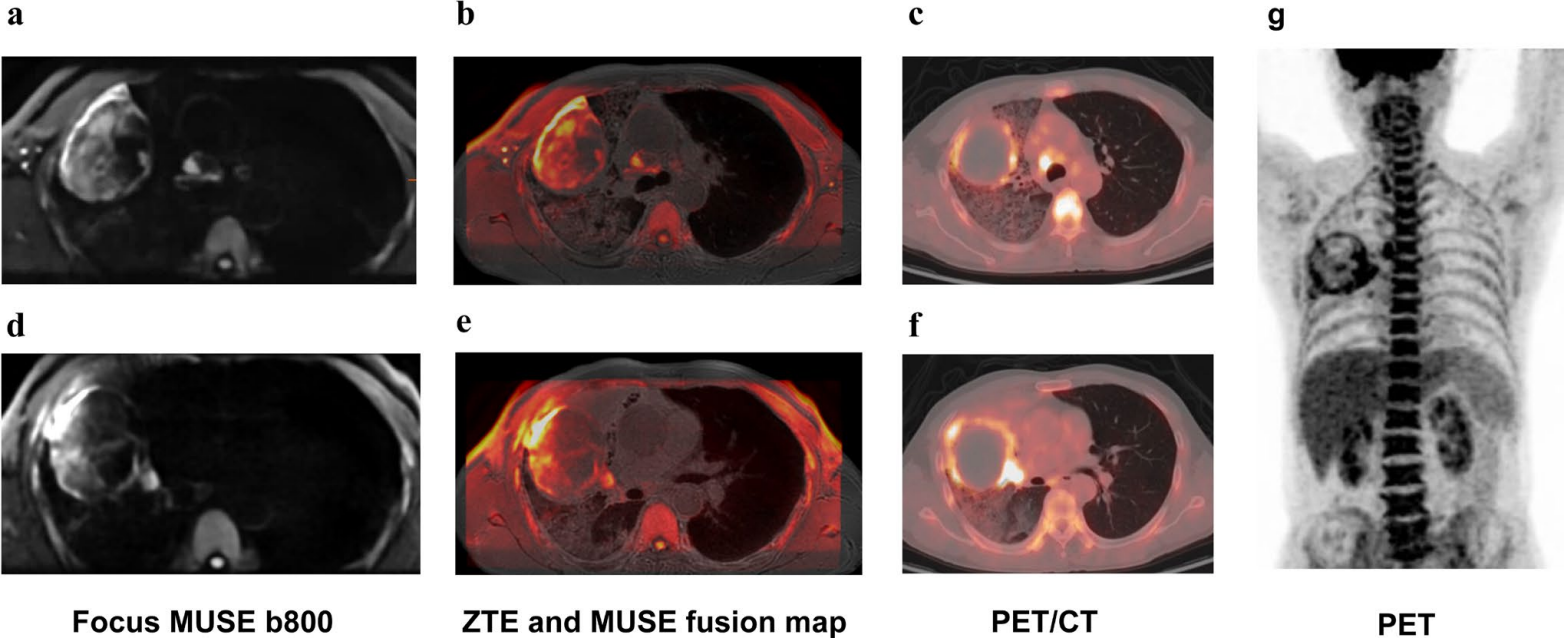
Male, 53 years old, lesion in the upper lobe of the right lung, compression atelectasis of the adjacent lung tissue, localized solid lesions, and enlarged lymph nodes visible in the mediastinum. (a, d) FOCUS MUSE DWI (*b* = 800); (b, e) ZTE and MUSE DWI fusion maps; (c, f, g ) 18F‐FDG PET/CT. The ZTE and MUSE DWI fusion maps show comparable performance to PET/CT imaging.
